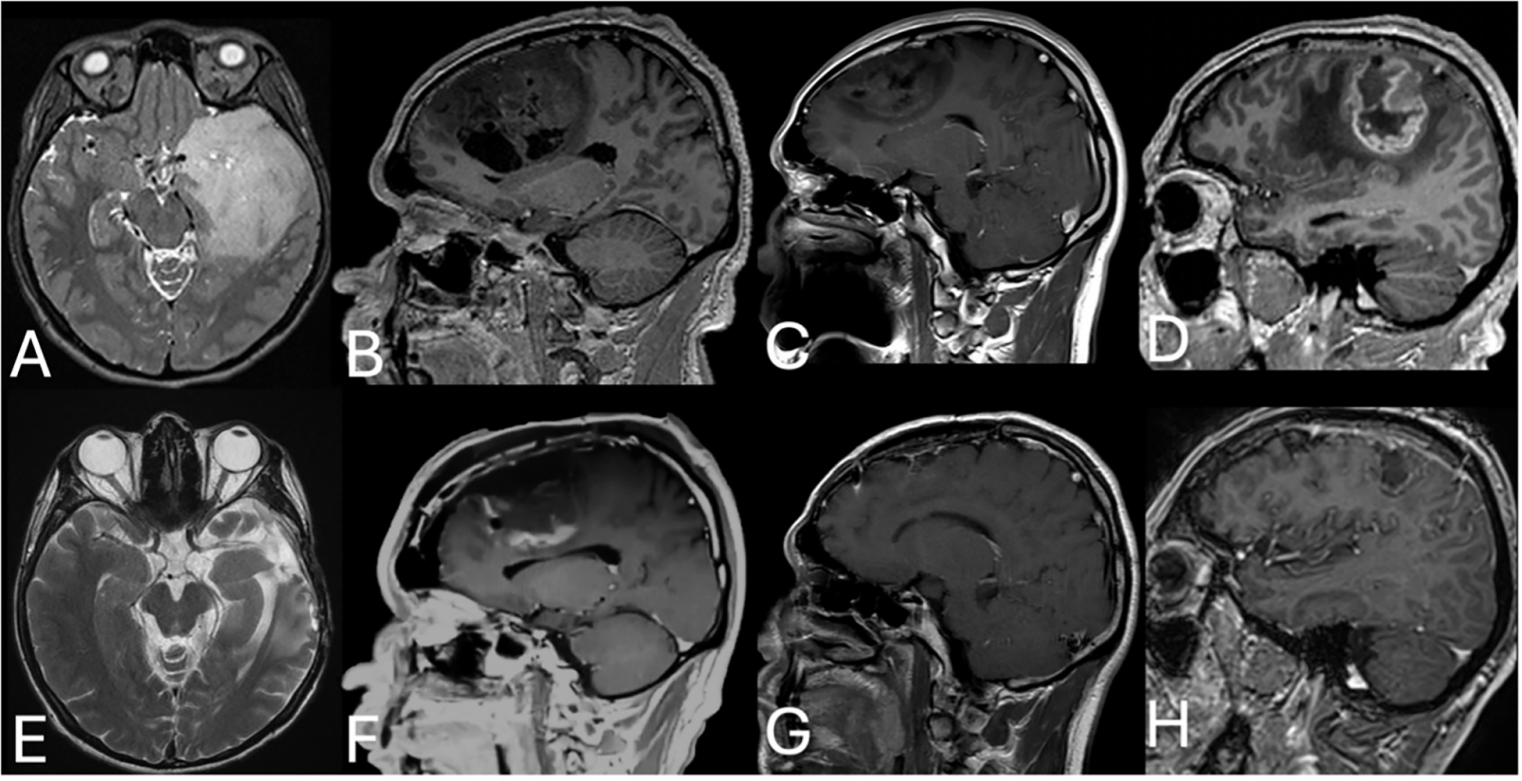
Pre- and postoperative MRI of diffuse gliomas. (A) Pre- and (E) postoperative MRI of a left temporal grade II astrocytoma. (B) Pre- and (F) postoperative enhanced MRI of a right frontal grade III oligodendroglioma. (C) Pre- and (G) postoperative enhanced MRI of a left frontal low-grade glioma (glioma, NOS). (D) Pre- and (H) postoperative enhanced MRI of a right perirolandic GBM.
